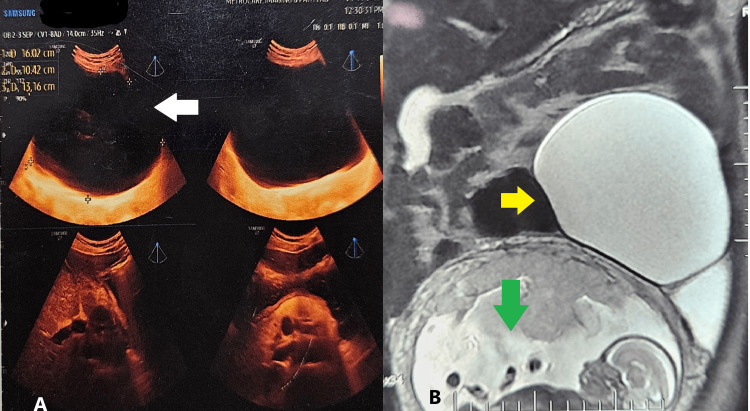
Radiological Investigation (A) Ultrasound: The white arrow points to a benign cystic structure. (B) Magnetic resonance imaging (MRI): The green arrow points to the fetus, while the yellow arrow highlights a hyperintense fluid-filled cystic lesion.
